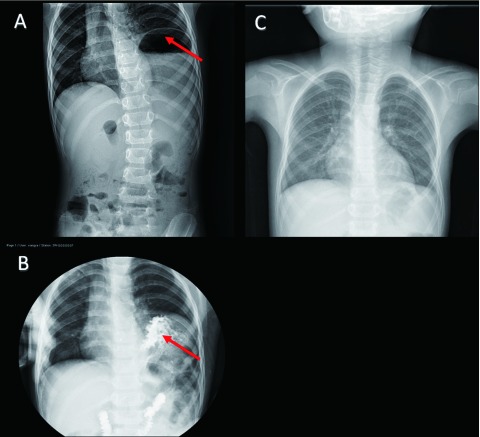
Preoperative plain abdominal radiograph upper gastrointestinal barium meal shows a raised left hemidiaphragm and gastric bubble (A & B). C is chest radiograph shows normal at follow-up of 3 years.
